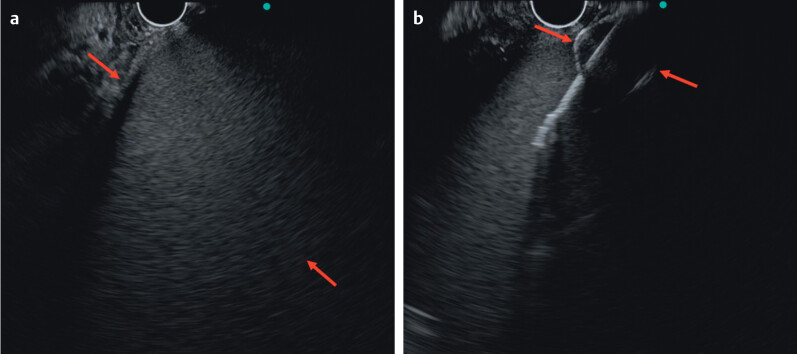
Endoscopic ultrasound (EUS) findings. (
